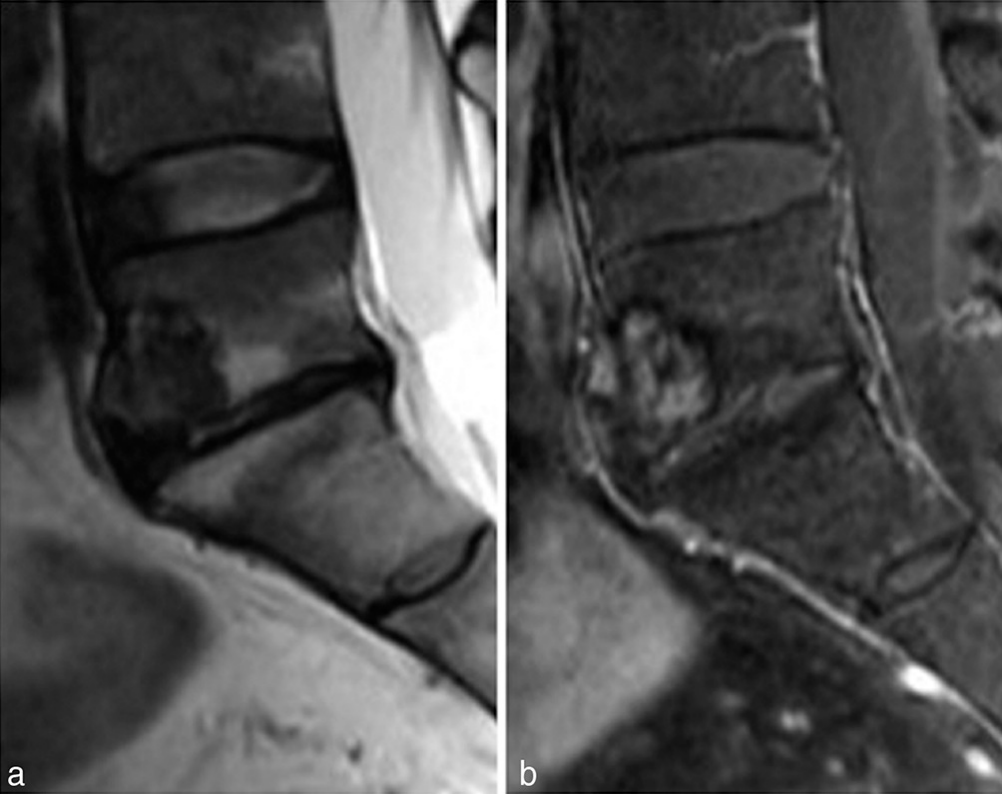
MRI of the lumbar spine. Pre-contrast *T*_2_ weighted images (a) reveal decreased signal intensity compared with the L5 vertebral body tumour. The tumour shows heterogeneous enhancement (b).
